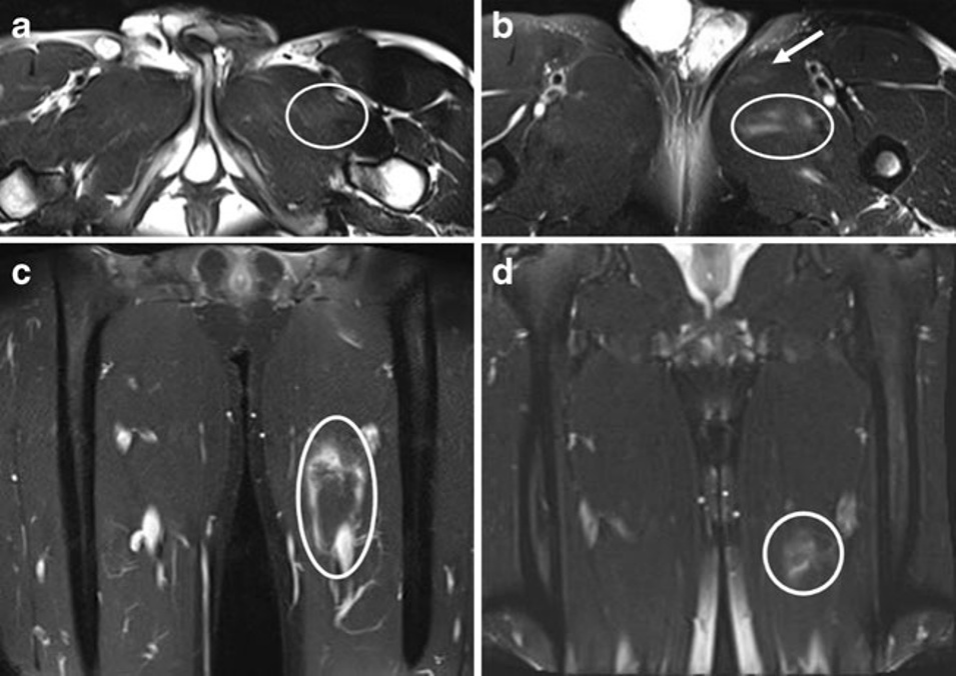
A 19-year-old gymnast presenting with an injury of the left groin. Axial FS T2-w TSE image shows (a) abnormal hyperintensity (*oval*) consistent with a strain of pectineus muscle, (b) discrete hyperintensity within the adductor longus (*arrow*, *strain without a tear*) and mild haematoma within the adductor brevis (*oval*, partial tear). (c) Haemorrhagic fluid extending into the fascia surrounding the adductor longus is shown (*oval*). The area of perifascial fluid spans a length of 10.8 cm over the proximal to mid aspects of the thigh. (d) The MR imaging at the 1-month follow-up showed some residual hyperintensity in the medial thigh (*circle*) corresponding with persistent oedema surrounding the adductor longus, but near-complete resolution of haematoma is noted. Normal homogeneous signal intensity was seen within the pectineus, adductor longus and brevis muscles, indicating recovery (not shown)
